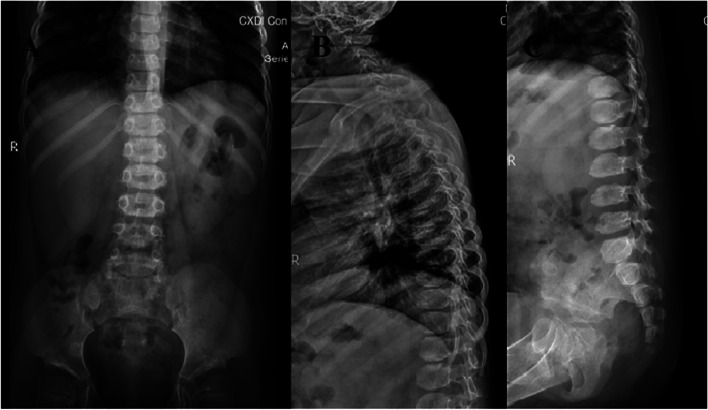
Anterior lateral radiograph of the spine of the patient. A concave proximal ends of the ribs, the irregular shape of the centrum and bilateral shallow acetabulum with irregular margin; (B) and (C) anterior beaking of the lower thoracic and lumbar vertebrae
